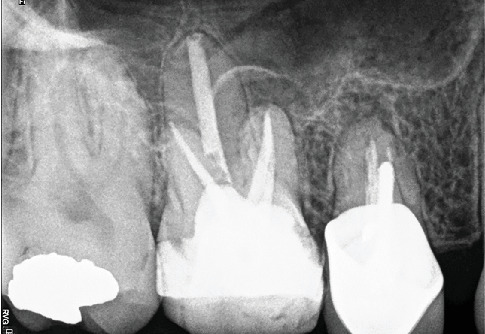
Pretreatment radiographic examination of Tooth #3 revealed the presence of an extensive periapical lesion and also dense obturating material in MB1, MB2, DB, and P canals.
